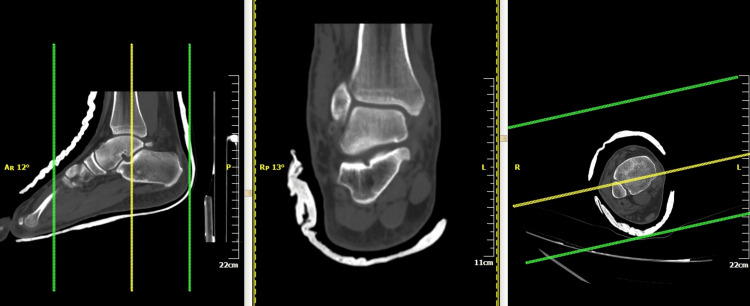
Post reduction CT scan of ankle was used to exclude associated fractures.
